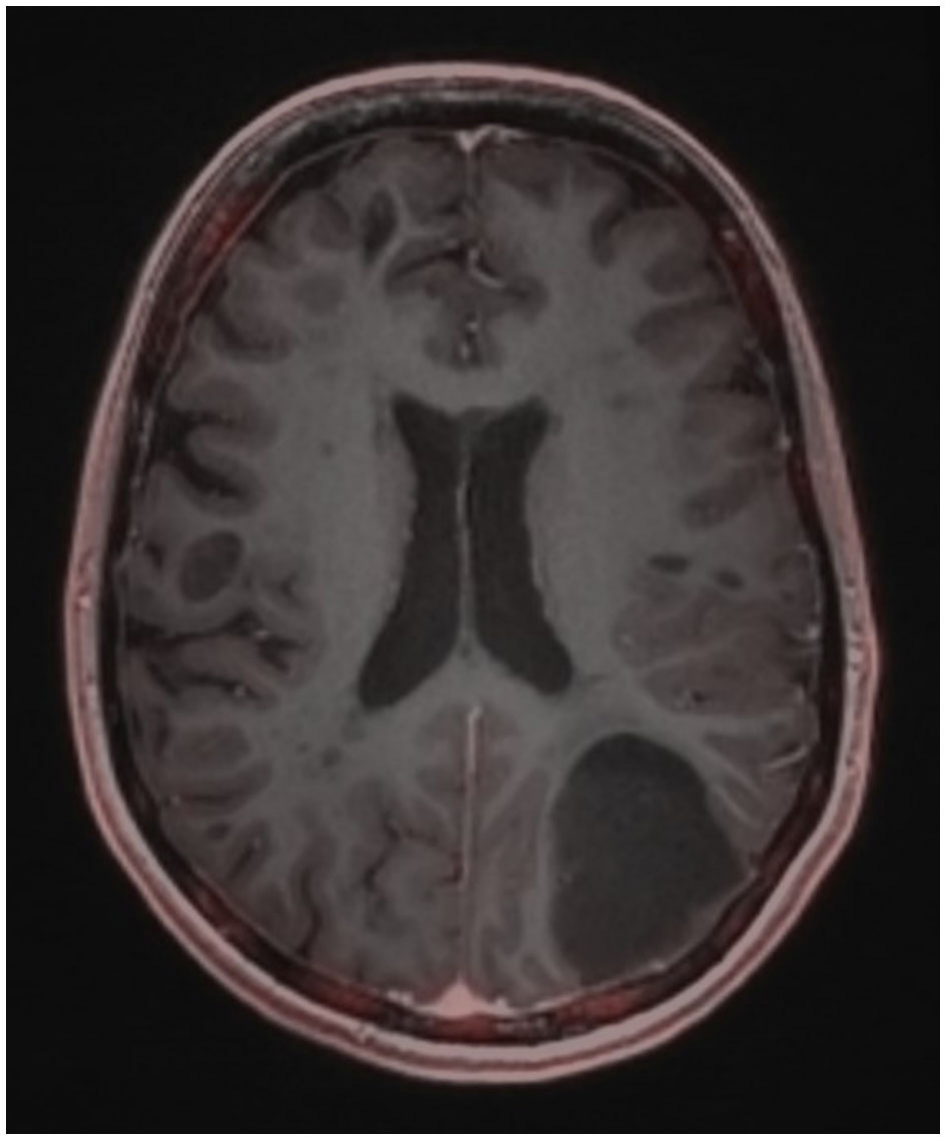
Brain MRI using contrast agents.
